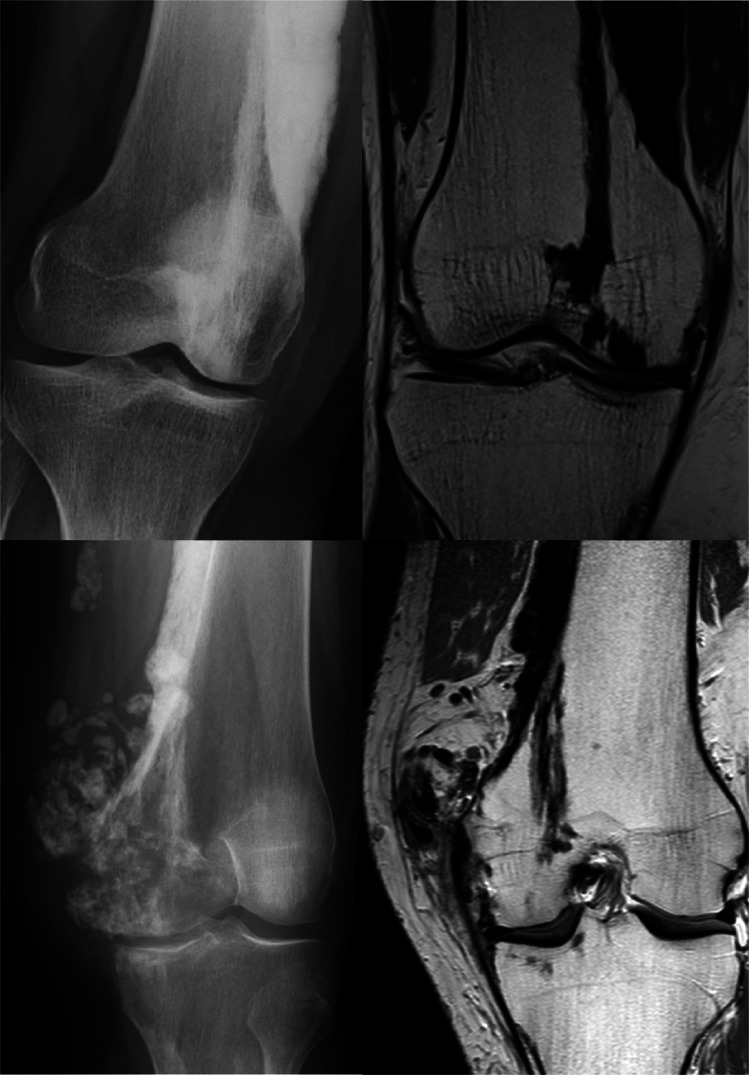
Forty-seven-year-old female with melorheostosis (top images). AP knee radiograph (top left) and coronal T1-weighted image (top right) reveal cortical and medullary hyperostosis with the medullary component appearing linear and striated, and more prominent in the epiphysis. Fifty-four-year-old male with melorheostosis (bottom images). AP knee radiograph (bottom left) and coronal T1-weighted image (bottom right) demonstrate a similar appearance, in addition to para-articular soft tissue involvement which (as in this case) is typically not attached to the underlying bone and can also contain fat or fibrovascular tissue. The appearances in both cases are consistent with a mixed sclerosing dysplasia, with additional features of osteopathia striata
